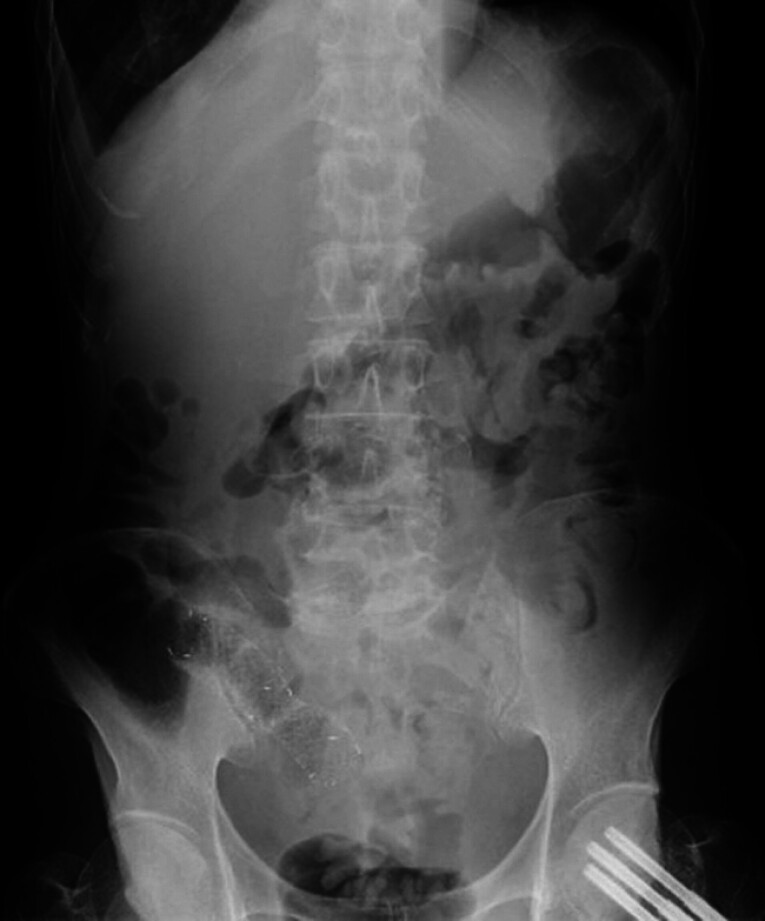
Radiographs taken 1 week post-stent placement, indicating improved bowel dilation and reduced gas accumulation.
